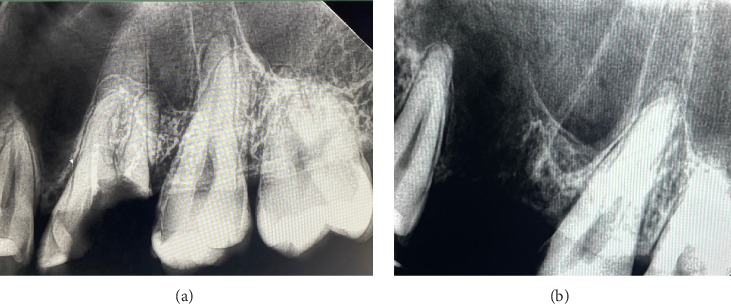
Periapical radiograph of Tooth 26: (a) before the extraction and (b) after the extraction.
